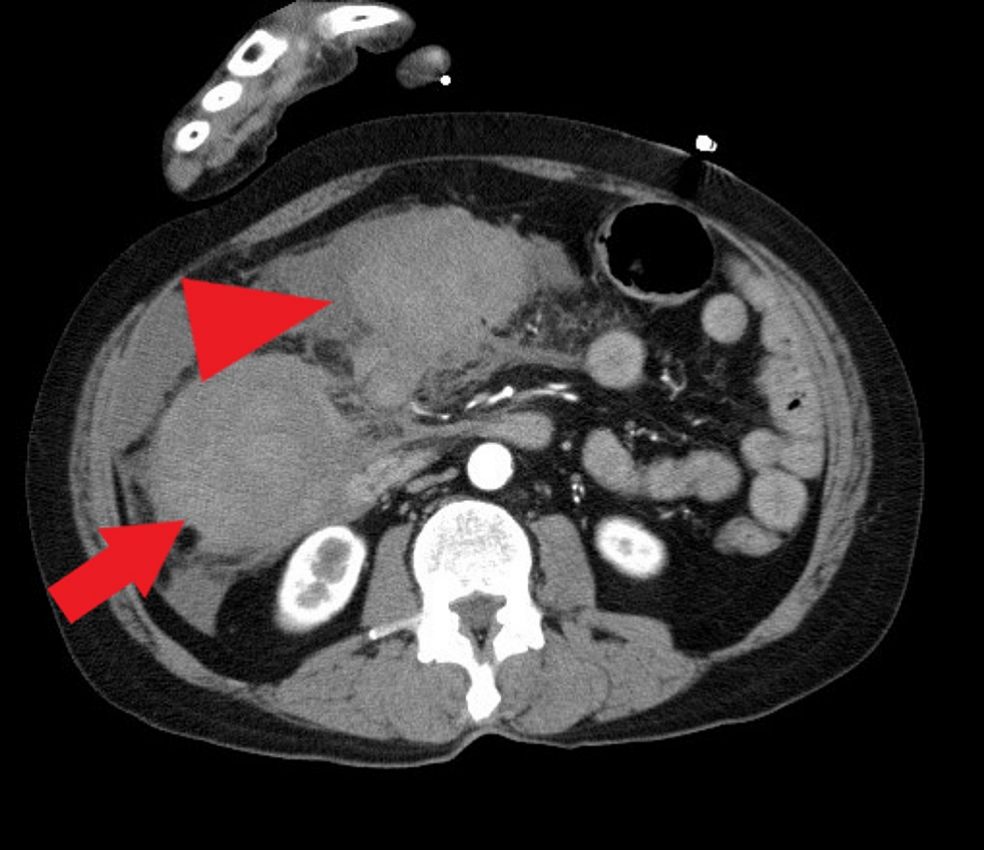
Preoperative contrast CT. Contrast CT showed hemorrhage ascites in the extrahepatic region (arrow) and hematoma in the omentum and omental bursa (arrowhead).
